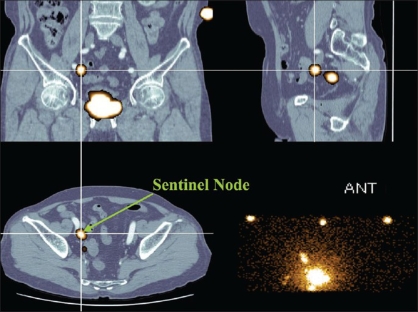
An SN is clearly indicated at the right external iliac region on CT-SPECT fusion image
